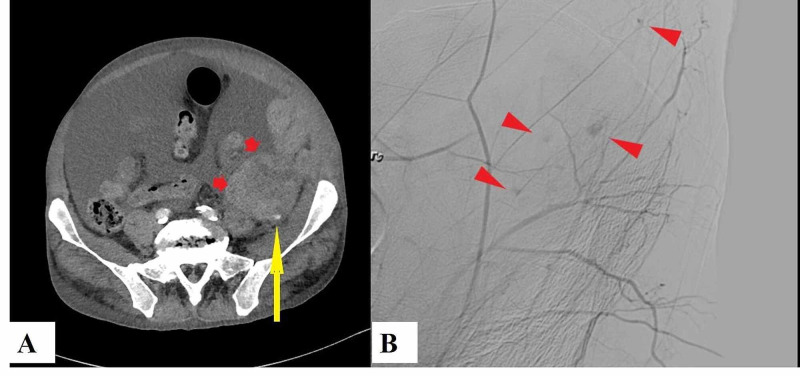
Contrast computed tomography scan showing extravasation (long arrow) around the retroperitoneal hematoma (short arrows) (A). Angiographic examination revealed multiple extravasations (arrowheads) in the peripheral vessels (B)
